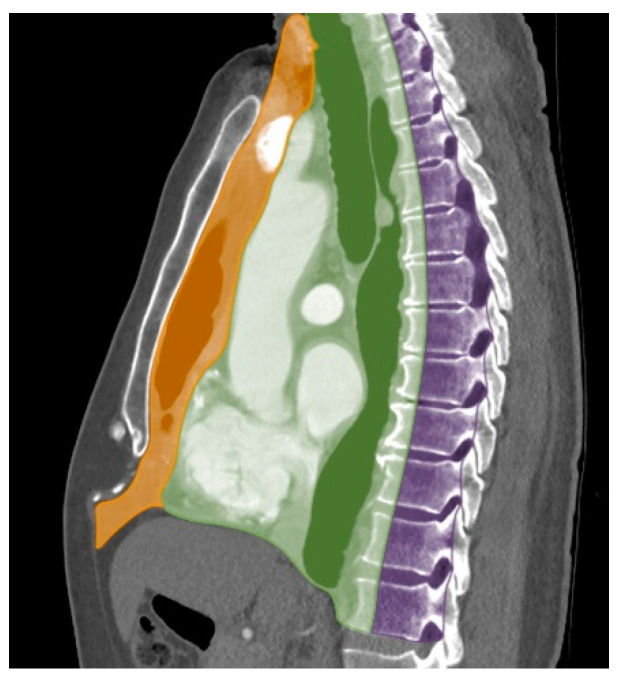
ITMIG definition of mediastinal compartments. Sagittal reformatted CT with color overlay shows mediastinal compartment borders: prevascular compartment (orange), visceral compartment (green) and paravertebral compartment (purple).
